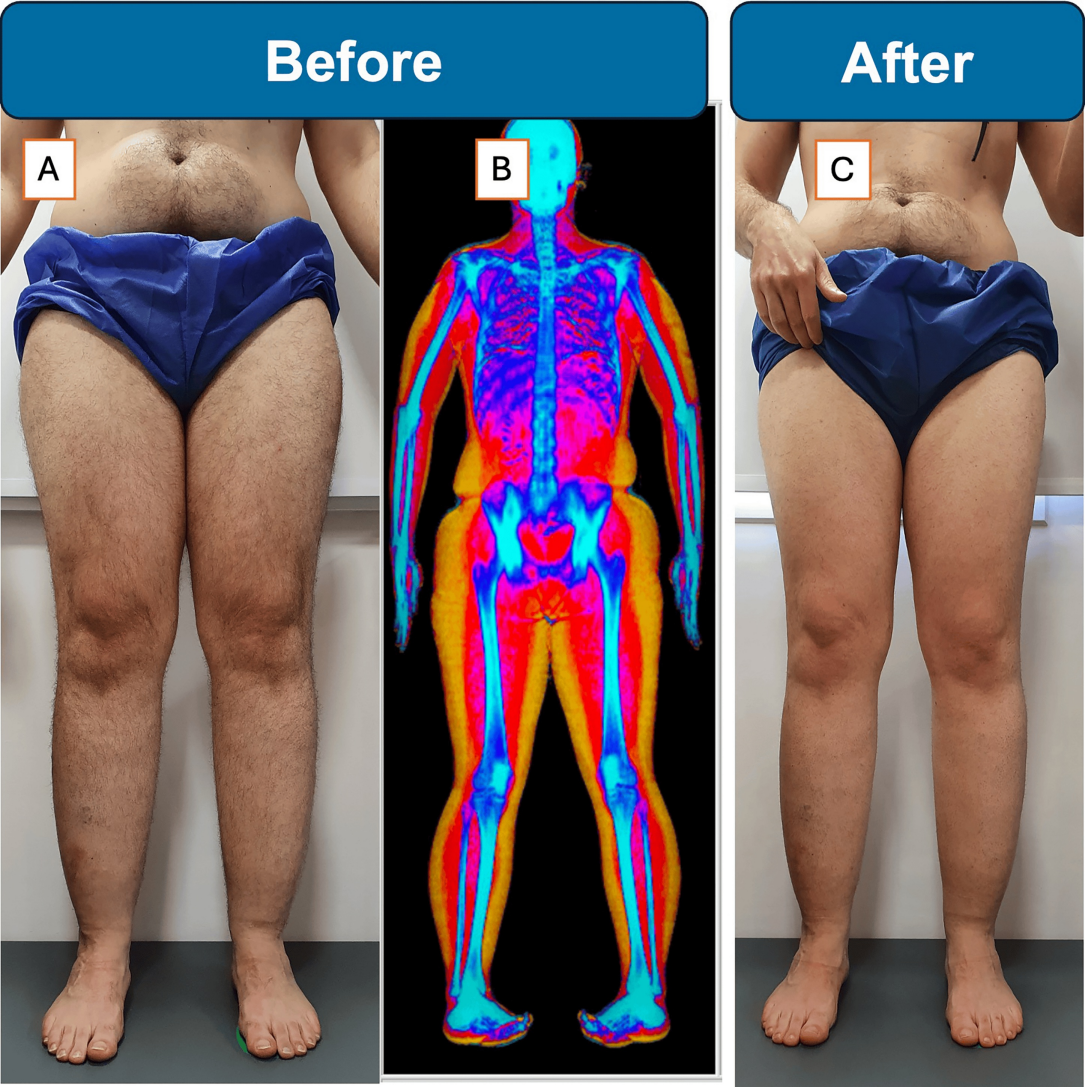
Case 3: before and after images with DXA fat distribution of a 44-year-old male with lipedema (A) Baseline anterior photograph demonstrating pronounced, symmetrical fat accumulation in the thighs and calves with sparing of the feet. (B) Dual-energy X-ray absorptiometry (DXA) map at baseline: warm colors indicate higher fat concentration in the lower extremities relative to the trunk. (C) Anterior photograph after four weeks of combined ketogenic diet, semaglutide, and lisdexamfetamine therapy, showing marked reduction in thigh girth and more uniform limb contour.
